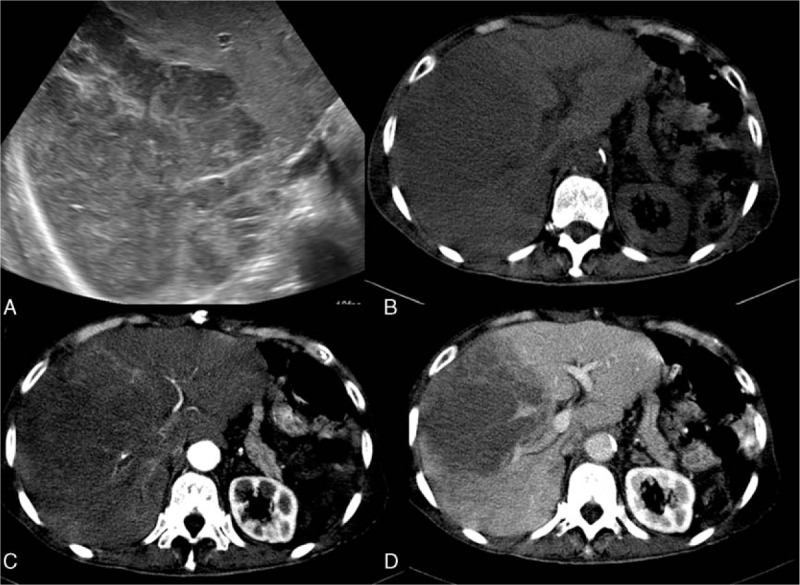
An 82-year-old man with suspicious primary lymphoma. No further involvement of other organs or marrow was noted 6 months after the diagnosis. A, Abdominal sonography shows a huge confluent hypoechoic mass at the right lobe of the liver. B, Precontrast CT. The mass shows relatively less enhancement than the surrounding liver parenchyma in both the arterial phase (C) and the delayed phase (D). Necrosis within the lesion is also depicted. CT = computed tomography.
